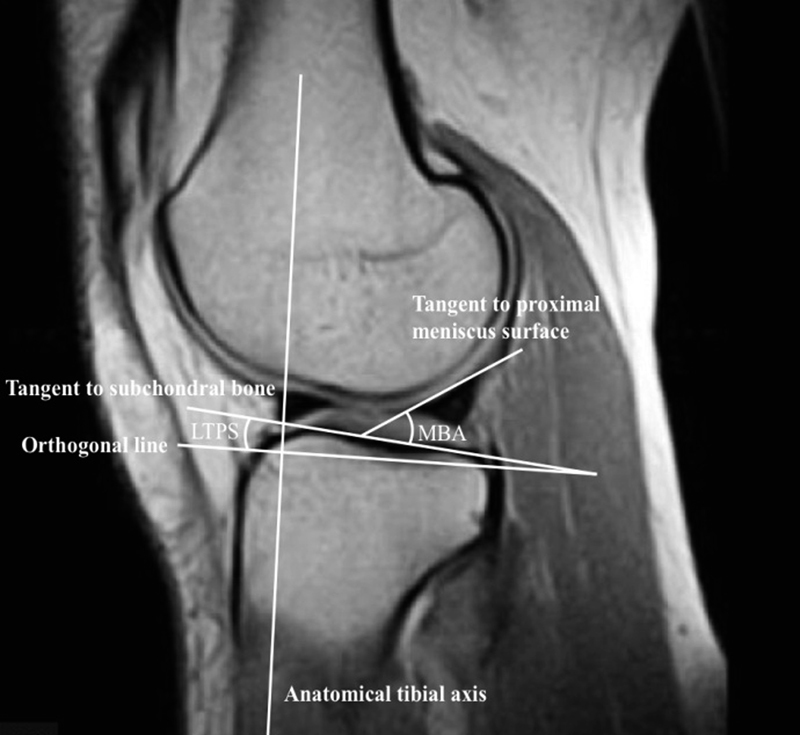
A tangent to the lateral plateau is drawn which connects the uppermost even part between the superior–anterior and posterior cortices. The angle between the orthogonal line to the magnetic resonance imaging (MRI) longitudinal axis and the tangent to the lateral plateau is defined as the lateral tibial posterior slope. The meniscal bone angle is measured as described by Sturnick et al
